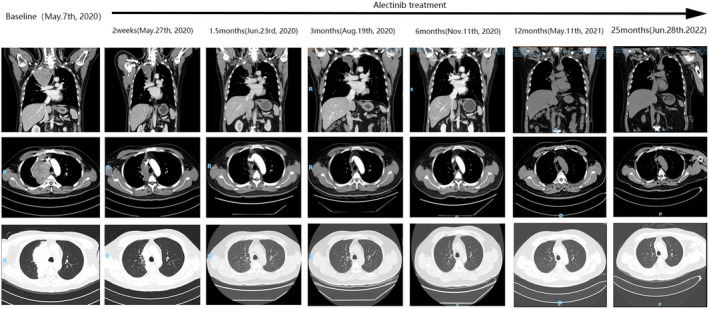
Significant reduction in the tumour volume was observed by the follow‐up CT scans from 2 weeks to 25 months post‐alectinib therapy. The most recent CT scan showed that the patient's lung tumour was close to disappearing, and he had almost achieved a clinical complete response (CCR).
